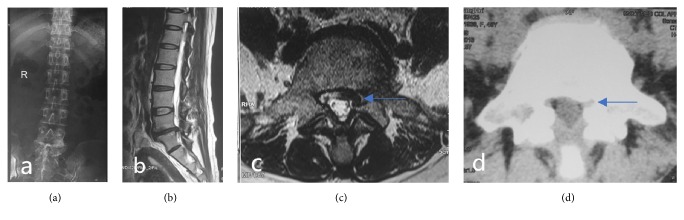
(a) Preoperative lumbar anteroposterior X-ray. (b), (c) Preoperative sagittal MRI T2-weighted images showing L_5_-S_1_ intervertebral disc degeneration and the lack of normal adipose tissue surrounding the left nerve root on the horizontal axis. Stenosis of the lateral crypt (arrows). (d) CT of the transverse axis of the lumbar intervertebral disc before surgery showing hyperplastic cohesion of the left facet joint and narrowing of the superior articular process (arrows).
